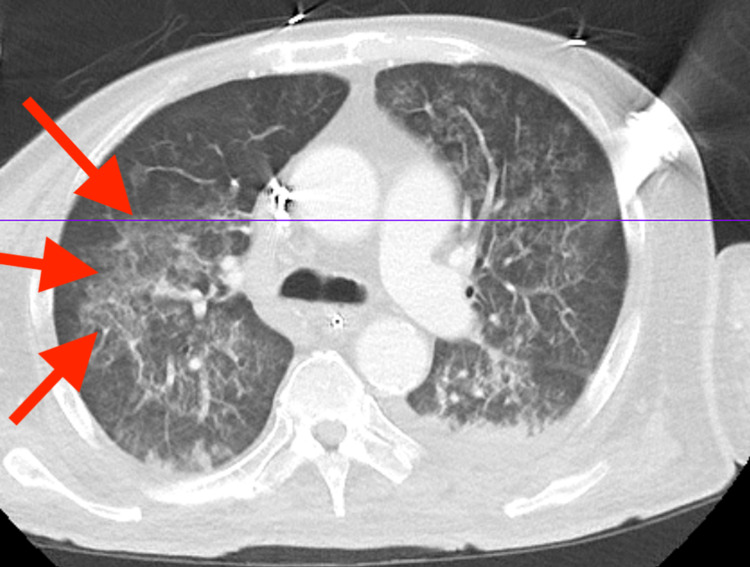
Abdominal and pelvic CT with contrast The image shows large mass-like opacity in the right upper lobe (arrows) with central small foci of air and necrosis that could represent extensive pulmonary consolidation and abscess formation versus mass, and diffuse bilateral ground-glass opacities with confluent areas of consolidation concerning for pneumonia versus pulmonary hemorrhage or edema CT: computed tomography
